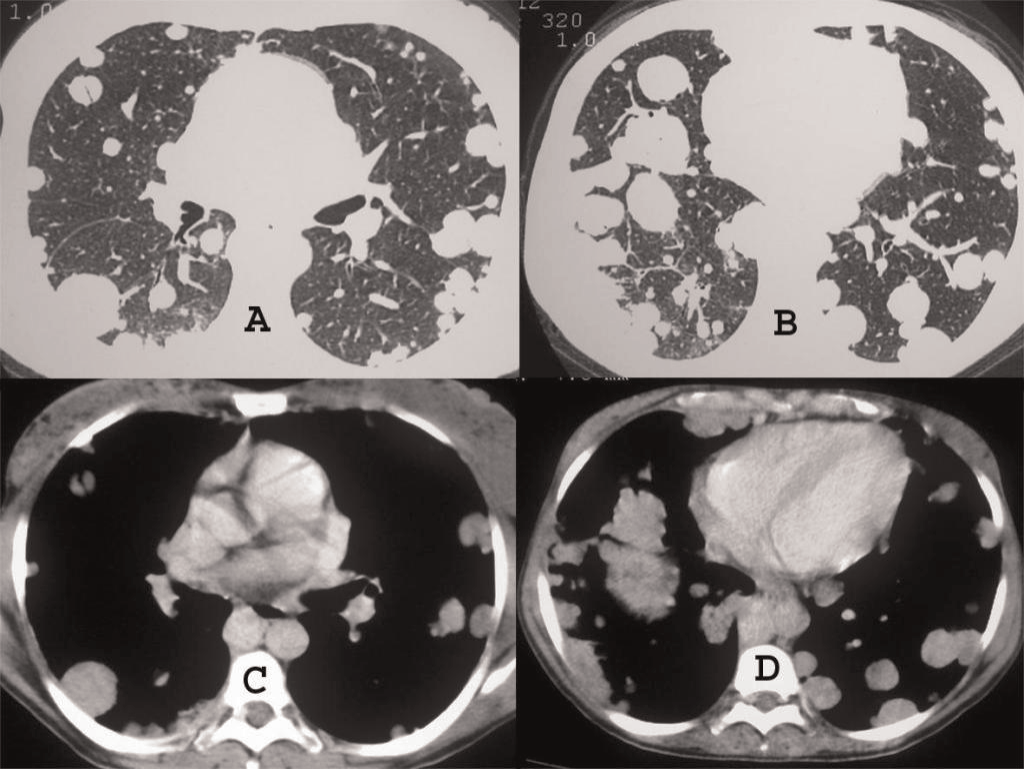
Computed tomography of the lungs, with pulmonary (A and B) and mediastinal (C and D) window settings, showing well-defined nodules, in a peripheral distribution.
